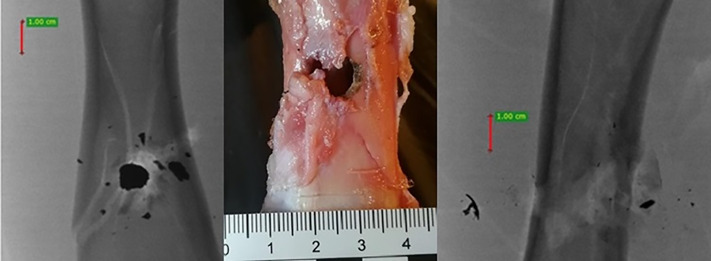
H&N Baracuda airgun pellet cal. 6.35 mm, on the left: A-P X-ray, centre: entry bone damage, on the right: lateral X-ray. Shot to the anterior surface of the femoral shaft.
